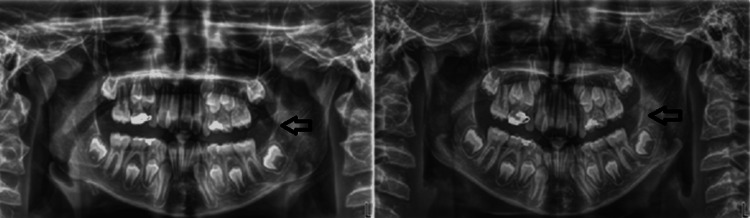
Preoperative orthopantomograms of the patient obtained with a three-month interval showing a relatively well-defined radiolucency at the left mandibular angle and ramus.
